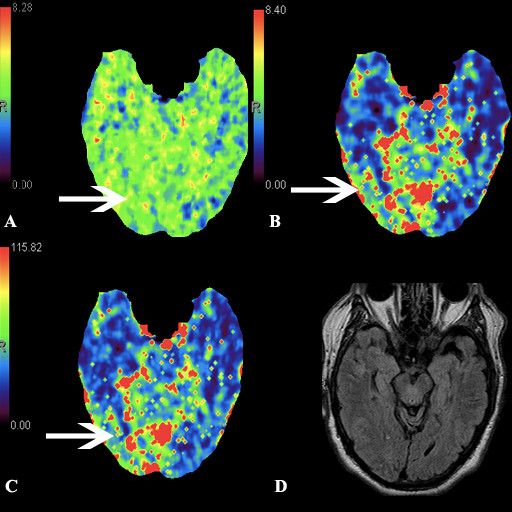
CT perfusion study demonstrating (a) shortened mean transit time in the right occipital territory (*arrow*). Corresponding area of increased cerebral blood volume (b) and increased cerebral blood flow (c). FLAIR MRI brain (d) without evidence of infarct.
